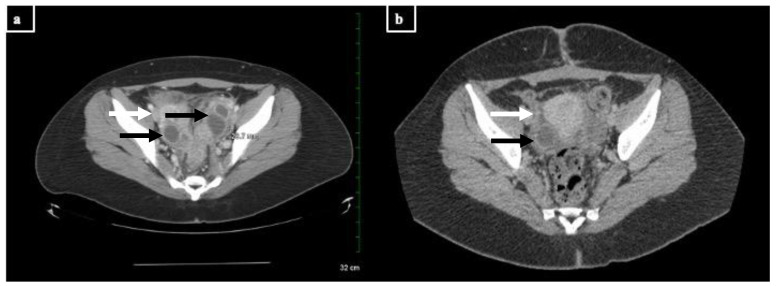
CT images of the TOAs before and after treatment with doxycycline and drainage (scale: 32 cm, measured dimension 28.7 mm at this level). Cystic structures are denoted with black arrows; inflammatory stranding is denoted with white arrows. (a) Representative image from the patient’s CT scan upon admission in March. There are multi-cystic structures with rim enhancement and inflammatory stranding in the bilateral ovaries consistent with bilateral residual or recurrent TOAs. (b) Representative image from the patient’s CT scan after treatment in June. There is a 2.0 cm benign cyst in the right ovary as well as moderate inflammatory stranding in the pelvis, consistent with recent pelvic inflammatory disease.
